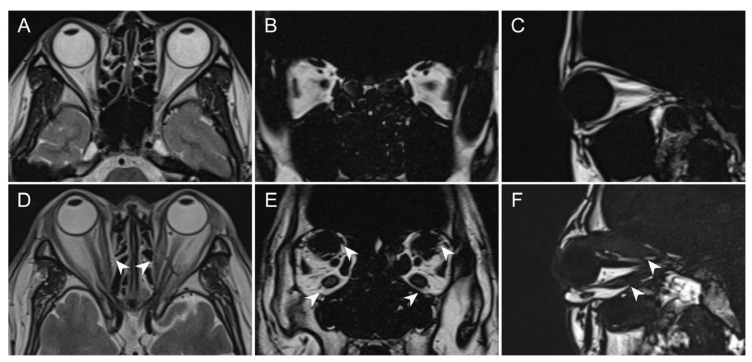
MRI findings comparing a healthy control and a DON patient. (A–C) Axial, coronal, and sagittal MRI images demonstrate normal orbital anatomy in the control subject. (D–F) Significant EOM enlargement is observed in all imaging planes of a DON patient (white arrowhead).
